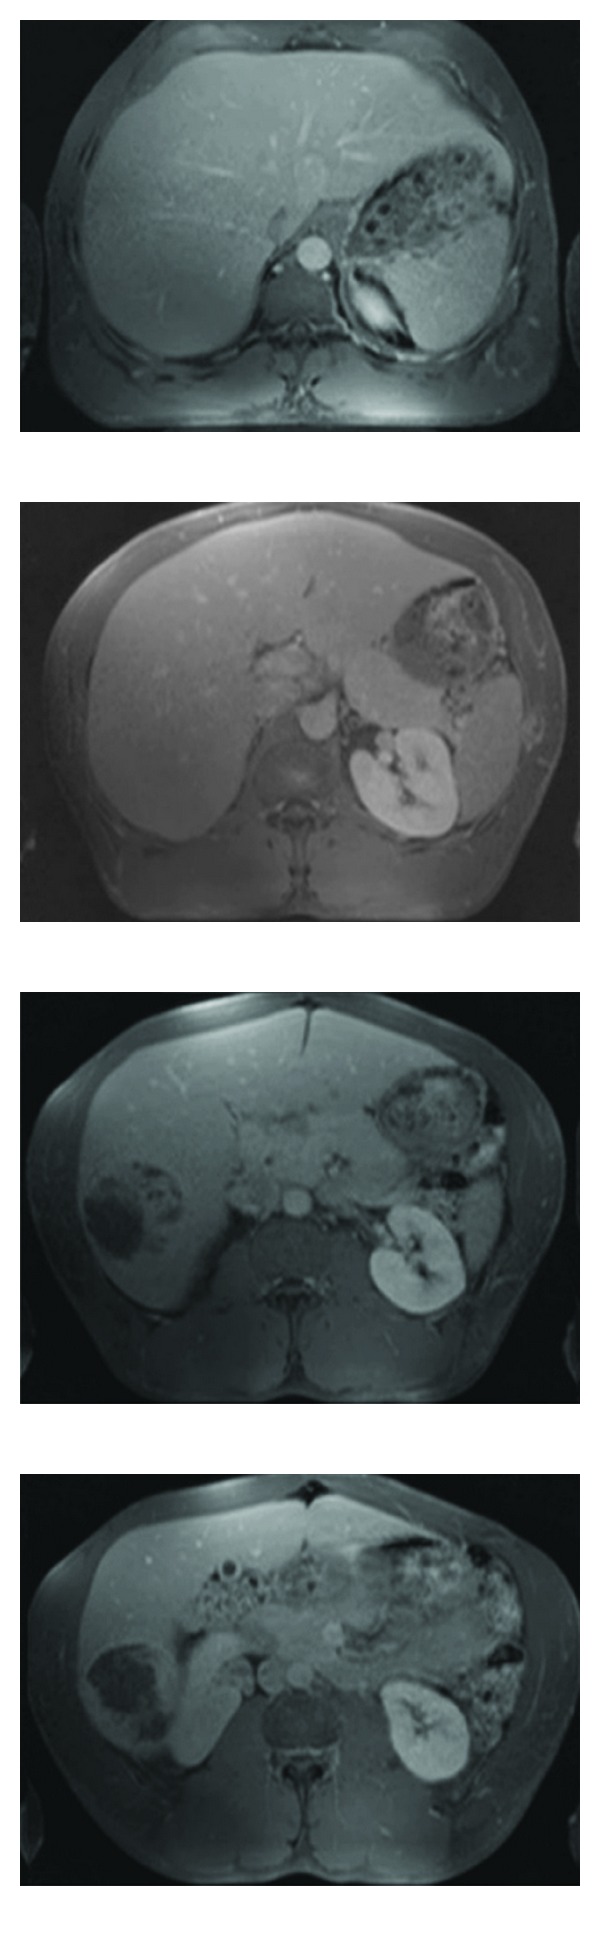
Magnetic resonance imaging showing the enlargement of the liver and the nonhomogeneous lesion in segment VI.
